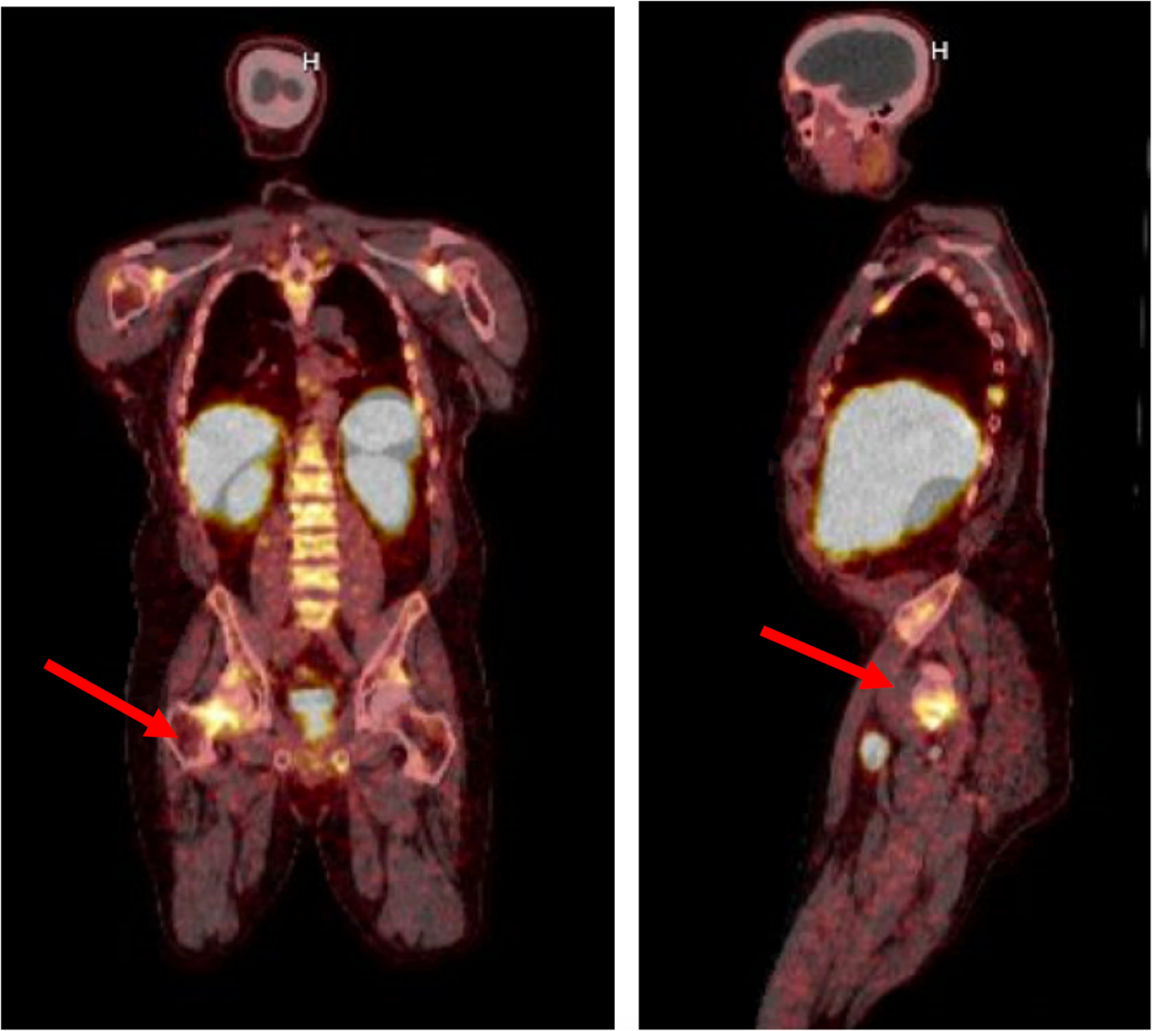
^68^Ga-DOTATATE PET/CT scan of patient in case 2 demonstrated marked tracer uptake in a 1.9 × 1.3 cm soft tissue mass underlying the right sartorius muscle (red arrows)
